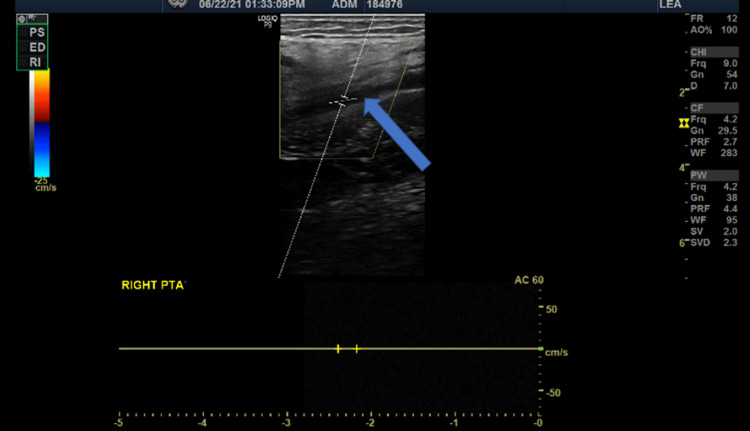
Doppler image shows absent blood flow in right posterior tibial artery (blue arrow)
